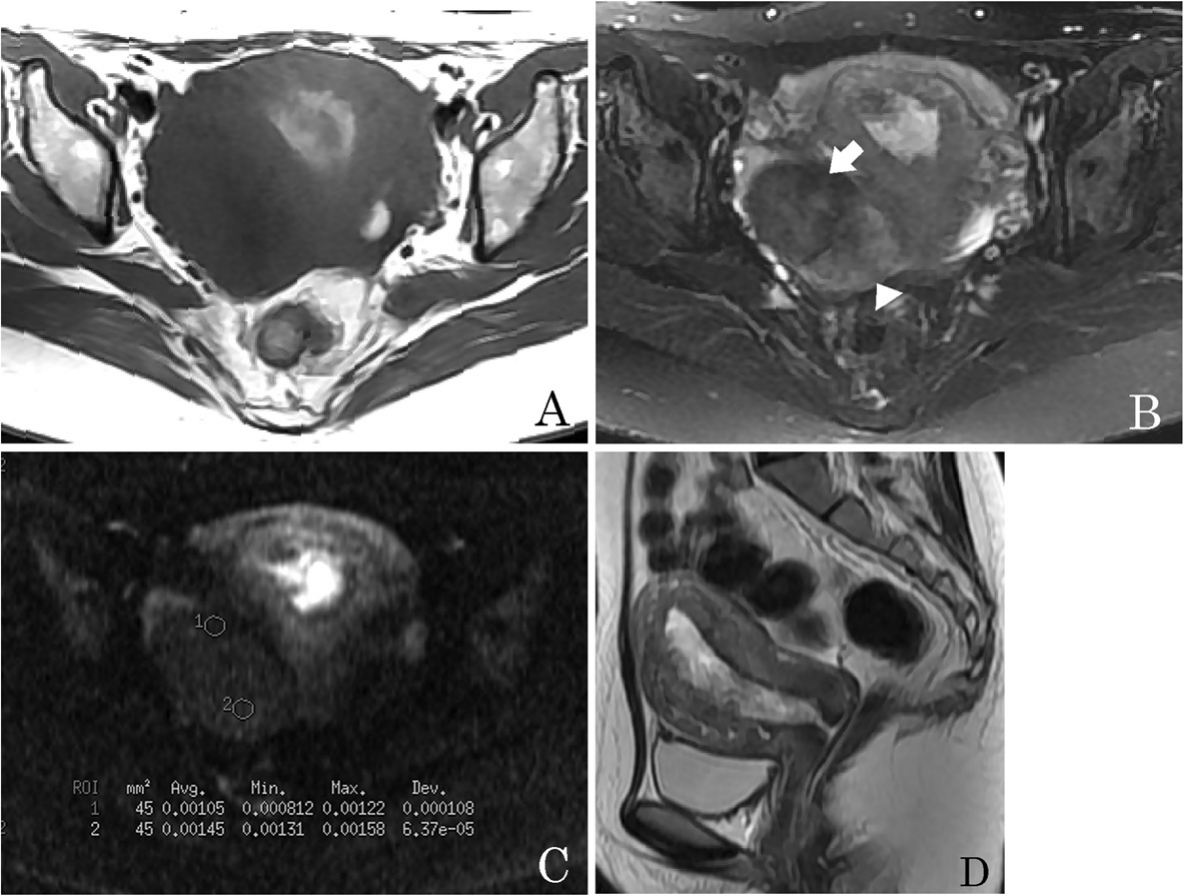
A 27-year-old woman with an ovarian fibrothecoma. (A) An axial T1-weighted image shows a right ovarian mass with hypointensity. (B) Axial T2-weighted image shows a solid heterogeneous signal mass; the area containing abundant fibrous tissue has marked low signal intensity (arrow) and the area containing an abundant thecoma component has intermediate signal intensity (arrowhead). (C) On axial diffusion-weighted imaging (DWI), the apparent diffusion coefficient (ADC) value of the fibrous tissue area is very low (circle 1, ADC = 1.05 × 10^-3^ mm^2^/s) and that of the thecoma component is very high (circle 2, ADC = 1.45 × 10^- 3^ mm^2^/s). (D) Sagittal T2-weighted image demonstrates endometrial thickening.
